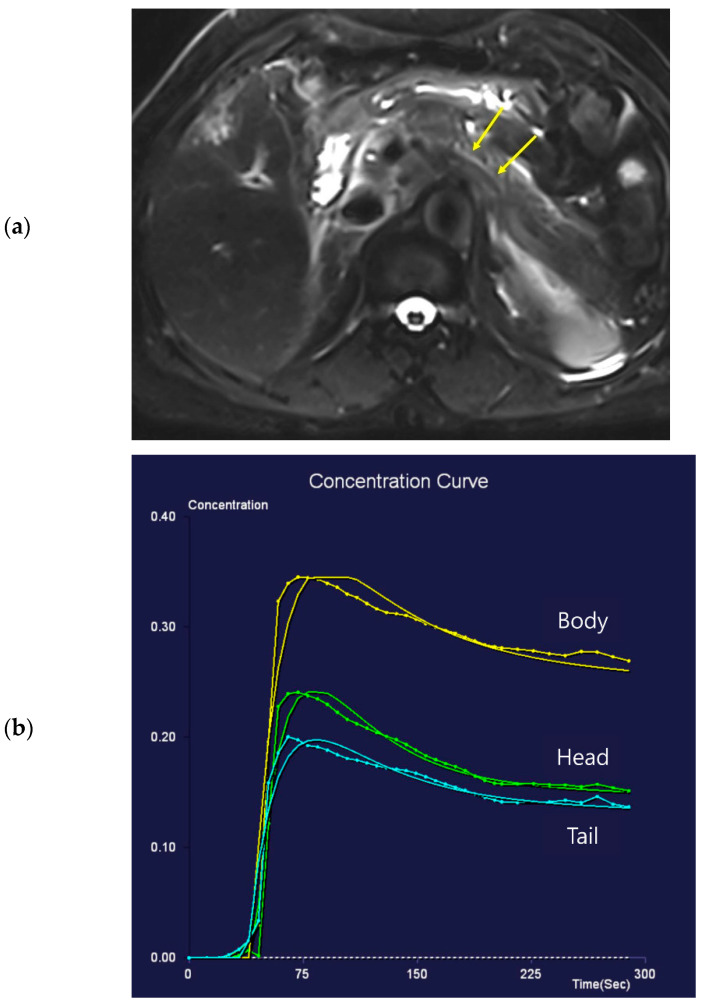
Seventy-one-year-old female patient undergoing an MRI to evaluate the gall bladder. There is no pancreatic dilatation (arrows) on the T2-weighted imaging (a) and no pancreatic focal lesion. Dynamic contrast-enhanced curves show rapid peak-enhancement and a gradual decrease in enhancement in the head, body, and tail (b).
